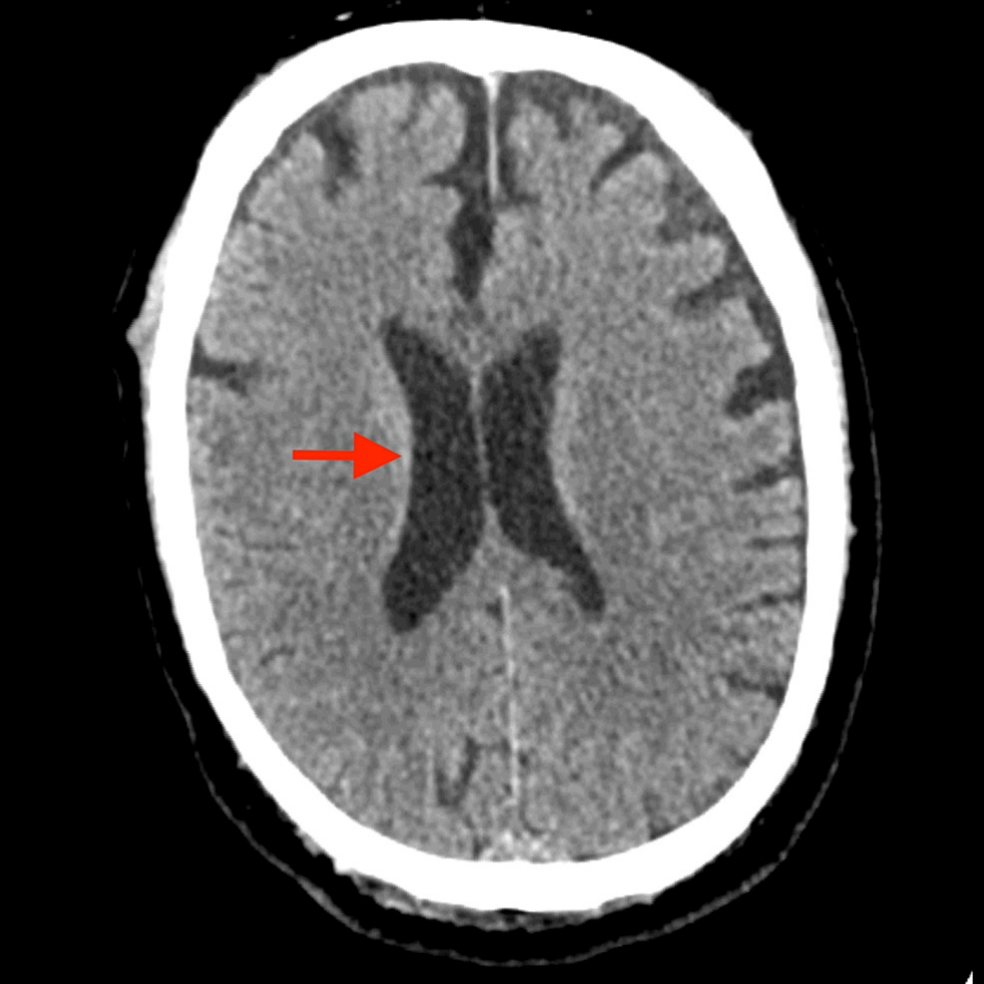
Axial section of cranial computed tomography scan Ventricular enlargement is characteristic of patients with Schizophrenia. It should be noted that there is no evidence of infection.
